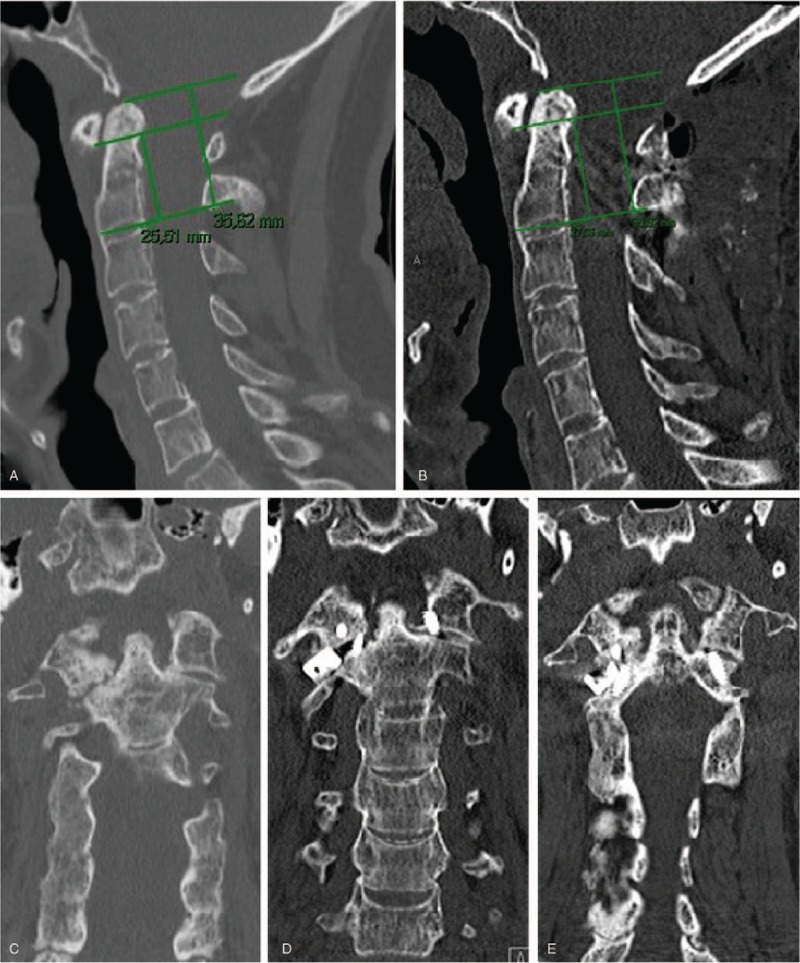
Images from case 3. (A) Preoperative sagittal CT image. The lines required to measure VAAI are shown. VAAI = 0.72. (B) Preoperative coronal CT image. The C1–C2 facet joint is severely damaged on the right side. (C) Postoperative sagittal CT image. The lines required to measure VAAI are shown. VAAI = 0.76. (D) Postoperative coronal CT image. The C1–C2 facet spacer is inserted on the right side and coronal alignment was improved. (E) Coronal CT image at 4 months follow-up. Callus formation and bony fusion in the right C1–C2 facet are present. CT = computed tomography, VAAI = vertical atlantoaxial index.
